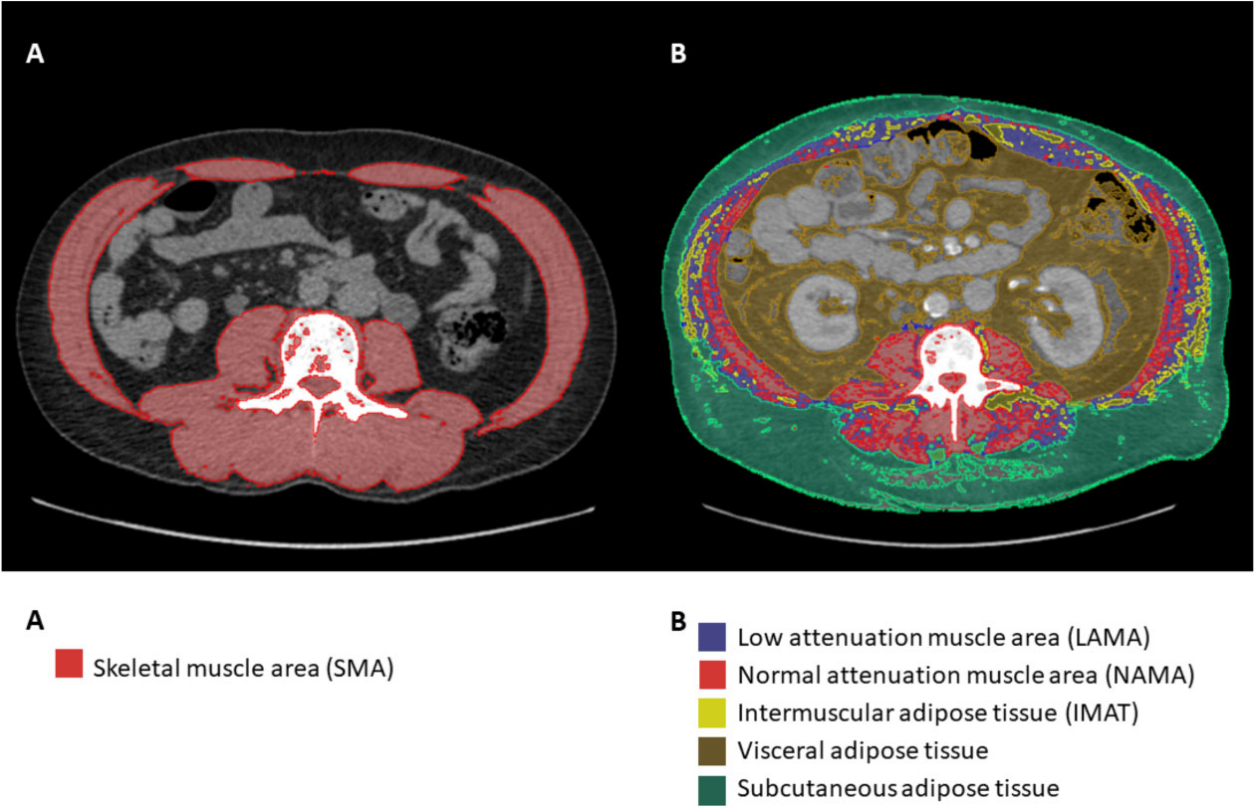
Body composition assessment by computed tomography (CT) at L3. (A) Skeletal muscle area at L3 using a range of −29–150 HU. (B) Different body compositions assessed by CT at L3.
